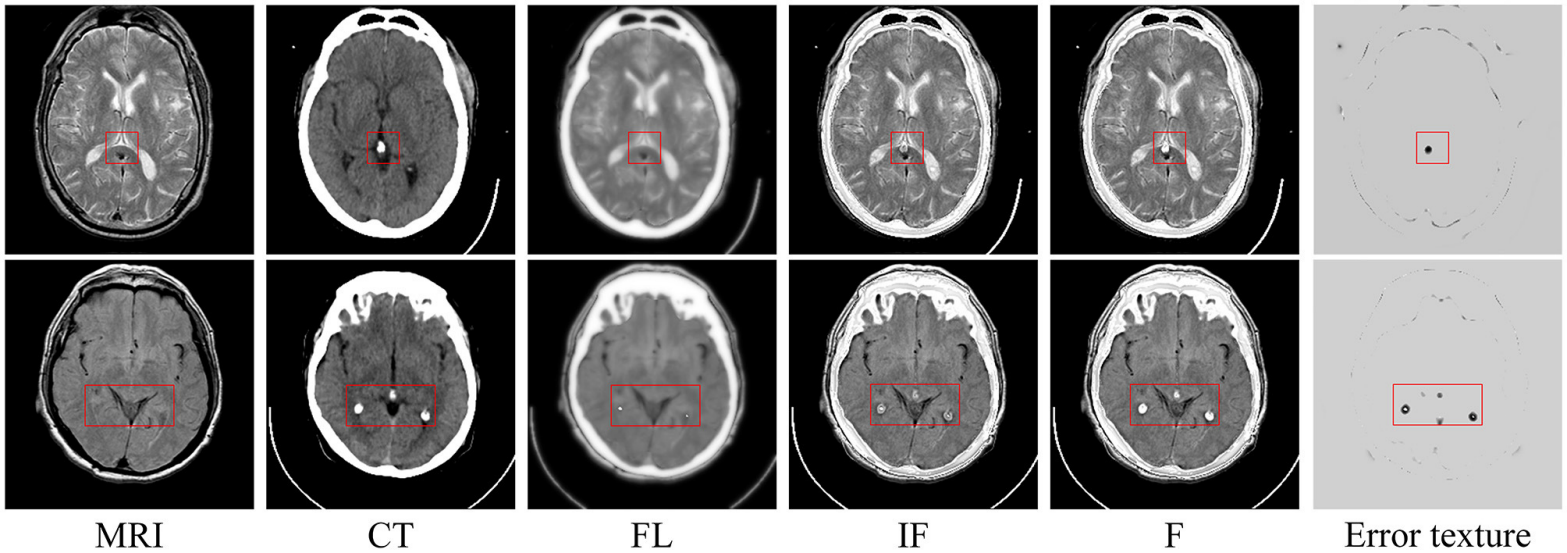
Experimental results of error texture removal strategy. Left to right: MRI image, CT image, fused low-frequency subbands, initial fusion results, final fusion results, and error texture frequency.
